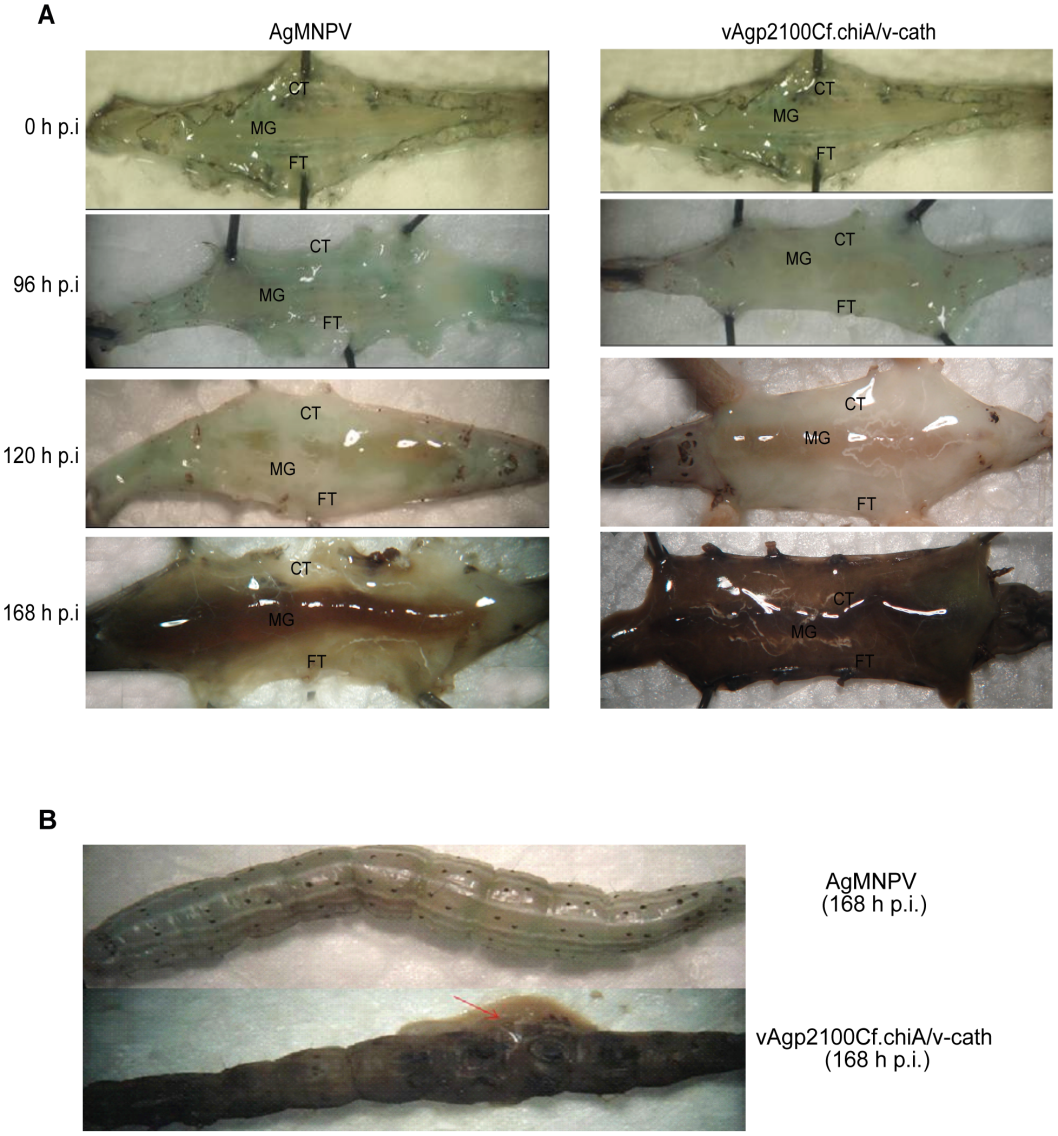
Effect caused by the wild type (AgMNPV) and recombinant (vAgp2100Cf.chiA/*v-cath*) viruses in *A. gemmatalis* larvae. (A) Structural analysis of internal tissues of larvae infected with AgMNPV and vAgp2100Cf.chiA/v-cath at different times post infection. MG – midgut, CT – cuticle; FT – fat tissue. (B) L. Larvae infected with wild type and recombinant virus at 168 h p.i., showing body liquefaction and melanized cuticle only in recombinant virus-infected larvae (vAgp2100Cf.chiA/v-cath).
